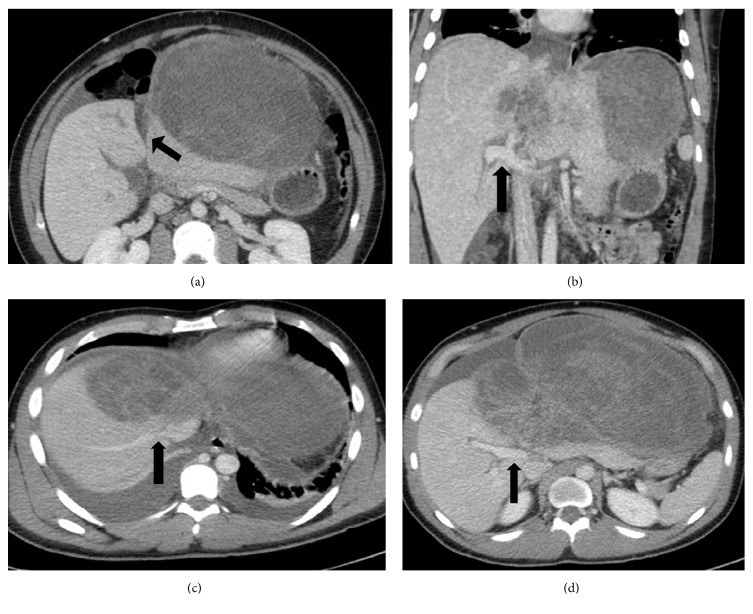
CT abdomen and pelvis with intravenous contrast showing large, heterogeneous left hepatic mass. Characterization of mass with regard to hepatic landmarks. (a) Arrow points to umbilical fissure. (b) Arrow identifies portal vein bifurcation. (c) Arrow points to juncture of right hepatic vein with inferior vena cava. (d) Arrow indicates right portal vein.
